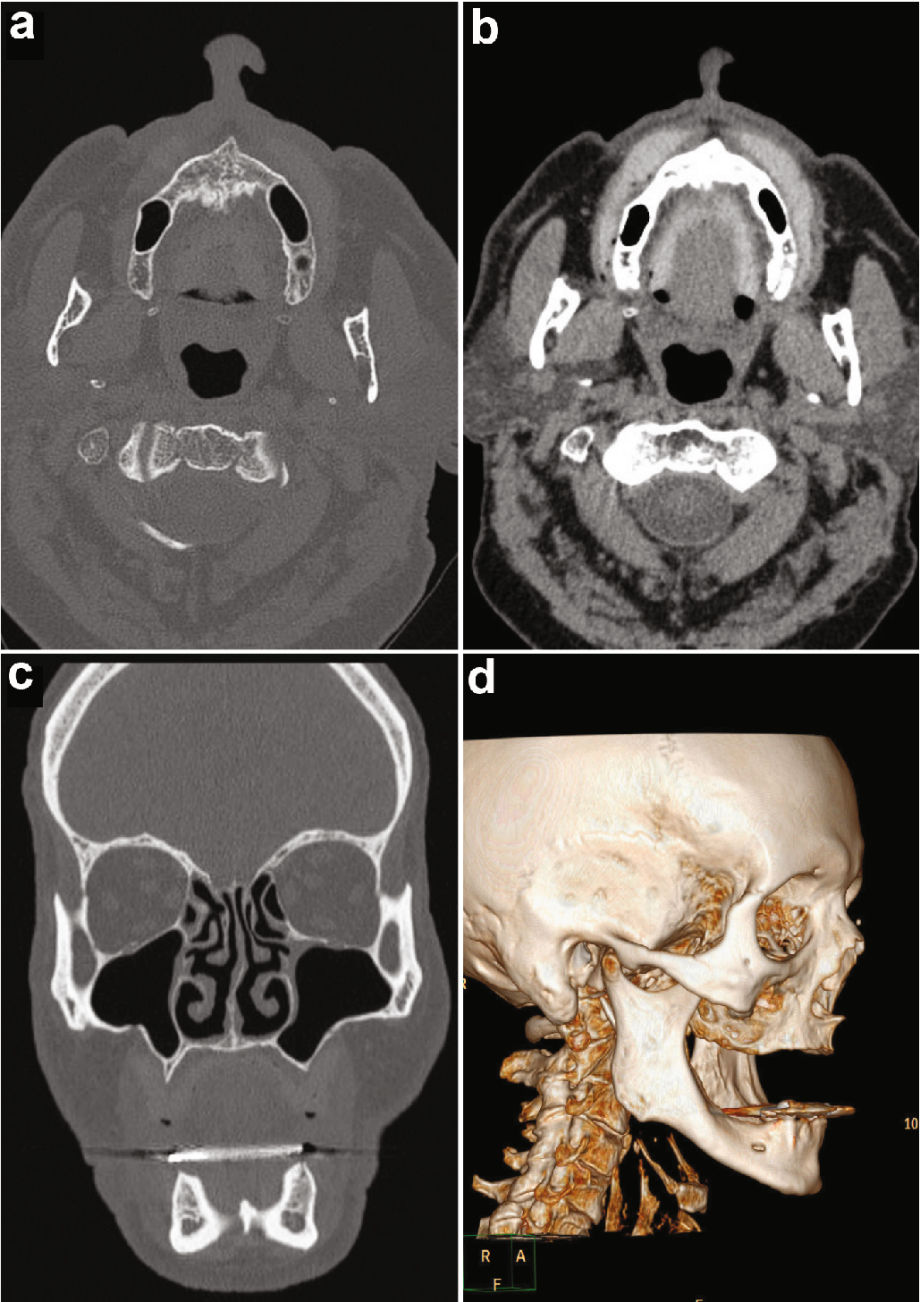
Multislice computed tomography examination of the patient showing no signs of the disease after lesion removal and the absence of other atherosclerotic changes in the head and neck. (a) Axial section of the upper jaw. (b) Soft tissue window of the axial section of the upper jaw. (c) Coronal section. (d) Three-dimensional reconstruction.
